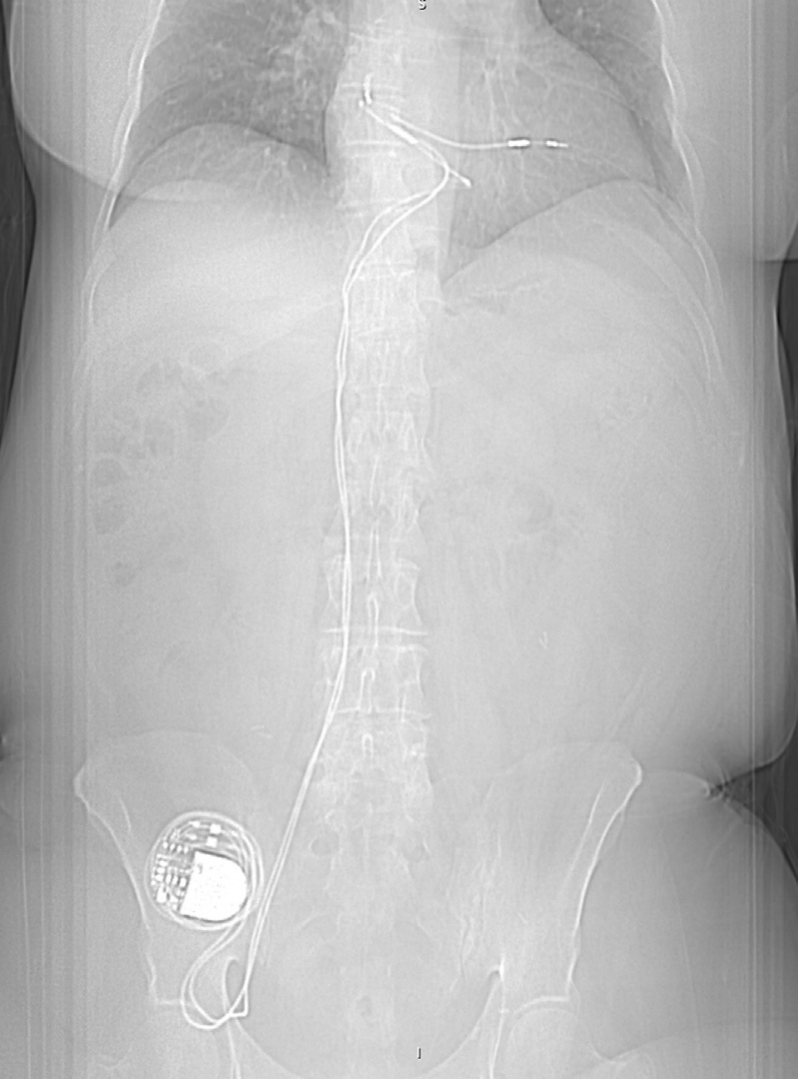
Radiographic image of permanent transfemoral dual-chamber pacemaker.
